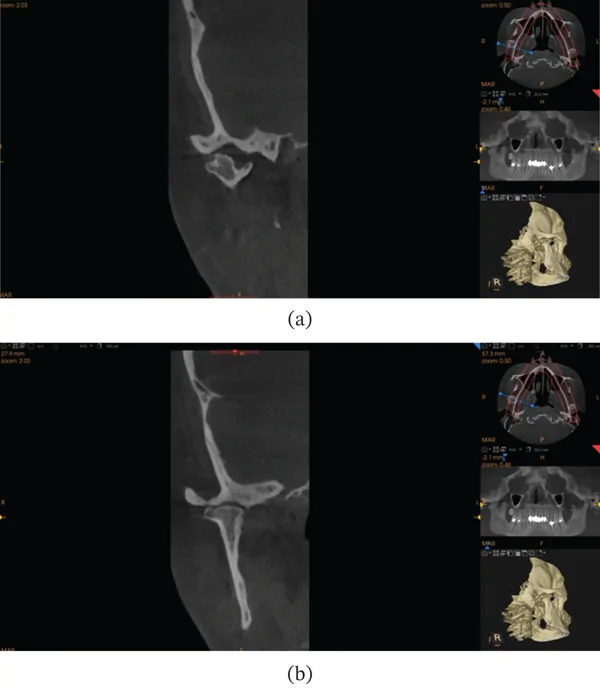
Sequential sagittal CBCT sections of the right mandibular condyle demonstrating the pentafid morphology. (a) Three distinct condylar heads are visible in the initial sagittal section, and (b) two additional heads appear in subsequent sagittal sections, confirming the presence of a total of five separate condylar heads.
